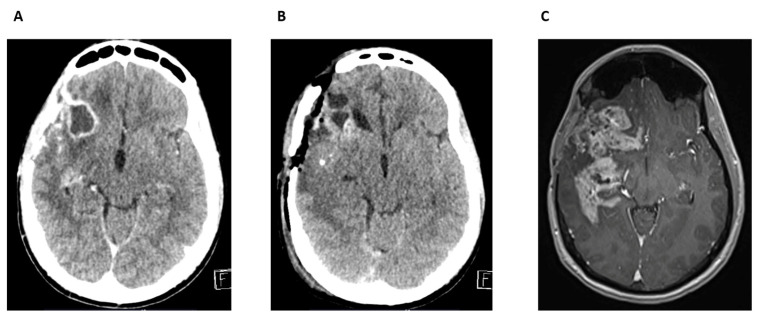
Cranial CT and MRI scan of patient 2. (A) Routine follow-up CT scan, axial view. Tumor recurrence in the right temporal region; (B) postoperative CT scan, axial view. Signs of infection; (C) routine MRI scan, axial slice. Signs of tumor progression.
